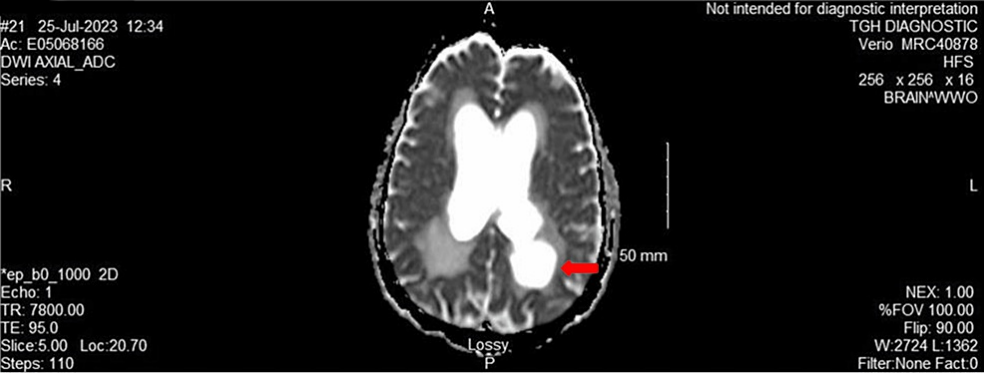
MRI brain: DWI axial ADC The image shows ex vacuo dilatation of the occipital horn of the left lateral ventricle ADC: apparent diffusion coefficient; DWI: diffusion-weighted imaging; MRI: magnetic resonance imaging
